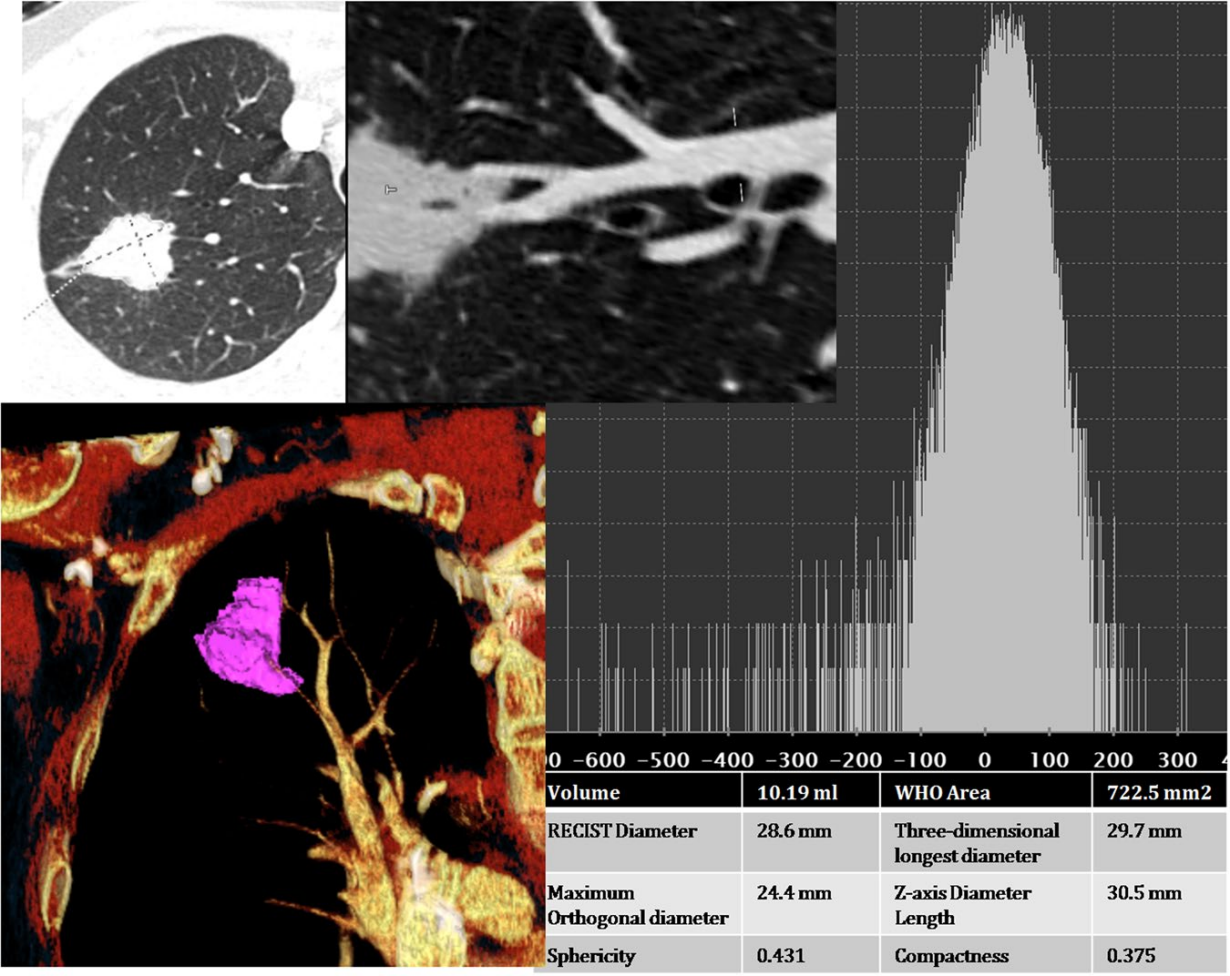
Example computed tomography (CT) images in a patient with stage IB lung adenocarcinoma. Axial longest diameter of the lesion was placed manually and the contour of entire-tumor volume of interest (VOI) was automatic segmented by LungCAD. Morphological features were extracted from the defined tumor contour on CT images.
